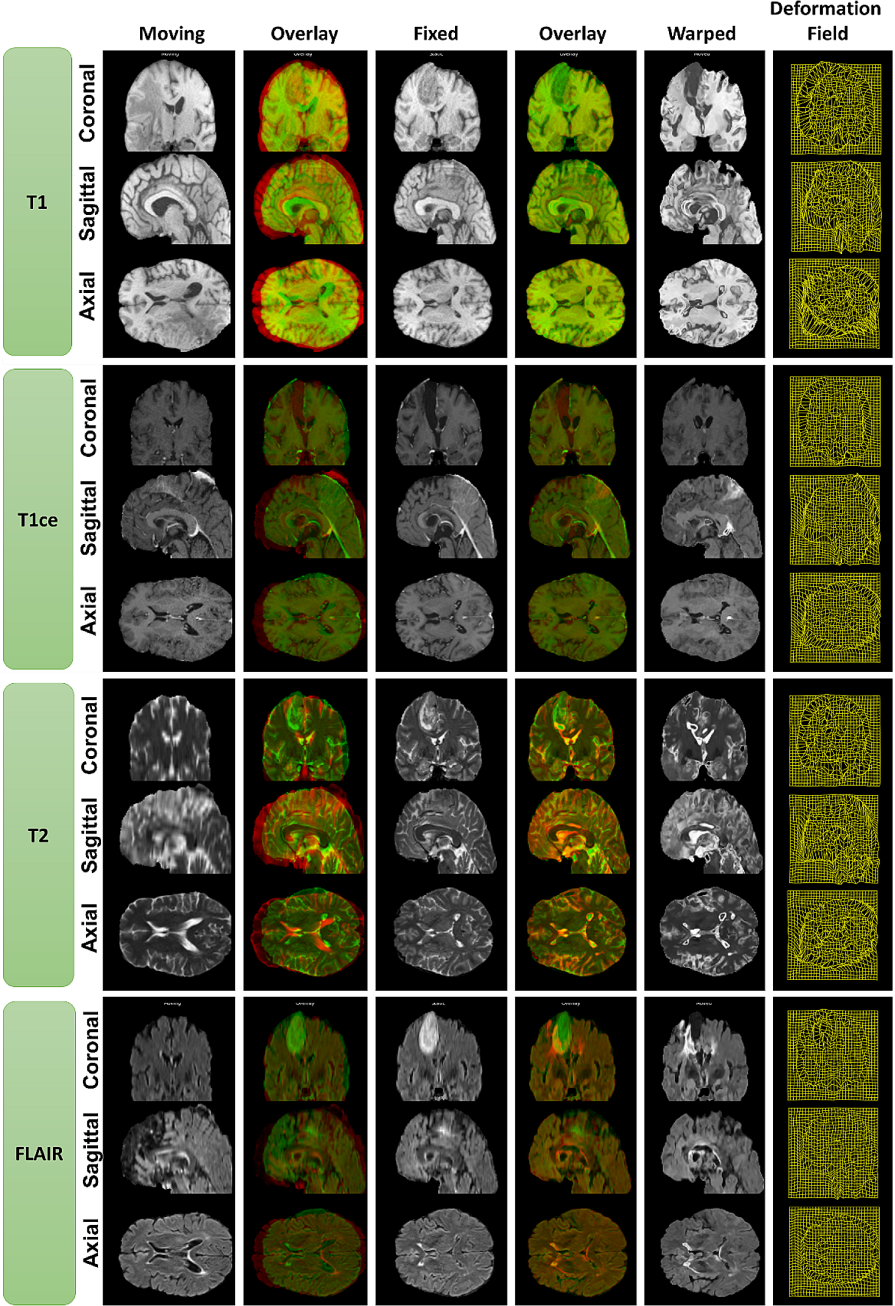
Example registration results of pairs of T1, T1-ce, T2, and FLAIR 3D MRI scans on three planes (axial, coronal, and sagittal) by our proposed ConvUNet-DIR model for one patient in the test set. Overlays of the fixed image (green color) and moving/warped image (red color) on top of each other and the deformation field are also displayed
